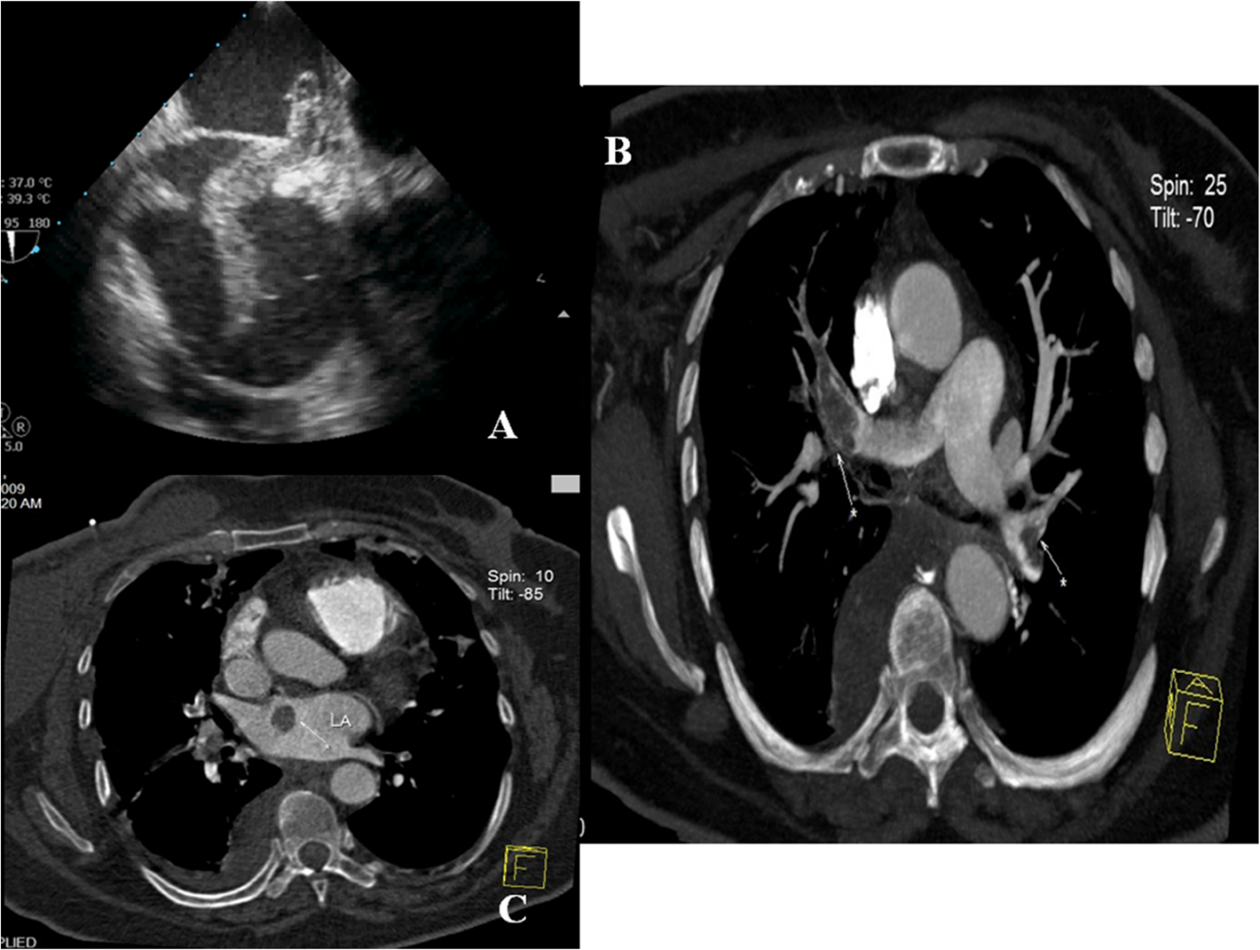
Pre-operative imaging studies. (A) Pre-operative transthoracic echocardiogram shows an entrapped thrombus in the foramen ovale. (B) Pre-operative contrast-enhanced computed tomographic scan shows a massive bilateral thromboembolism (white arrows). (C) Pre-operative contrast-enhanced computed tomographic scan shows the intracardiac thrombus (white arrow).
